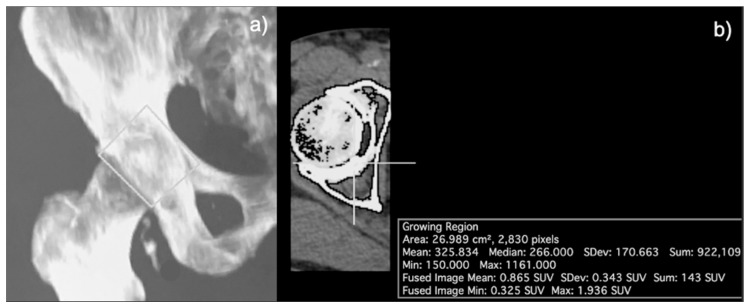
Region of interest (ROI) of the hip joint. (a) Coronal view of the CT segmentation showing the rectangular region around the head of the femur and its articulation with the acetabulum, excluding the femoral neck. (b) Axial view depicting the morphological closing process.
